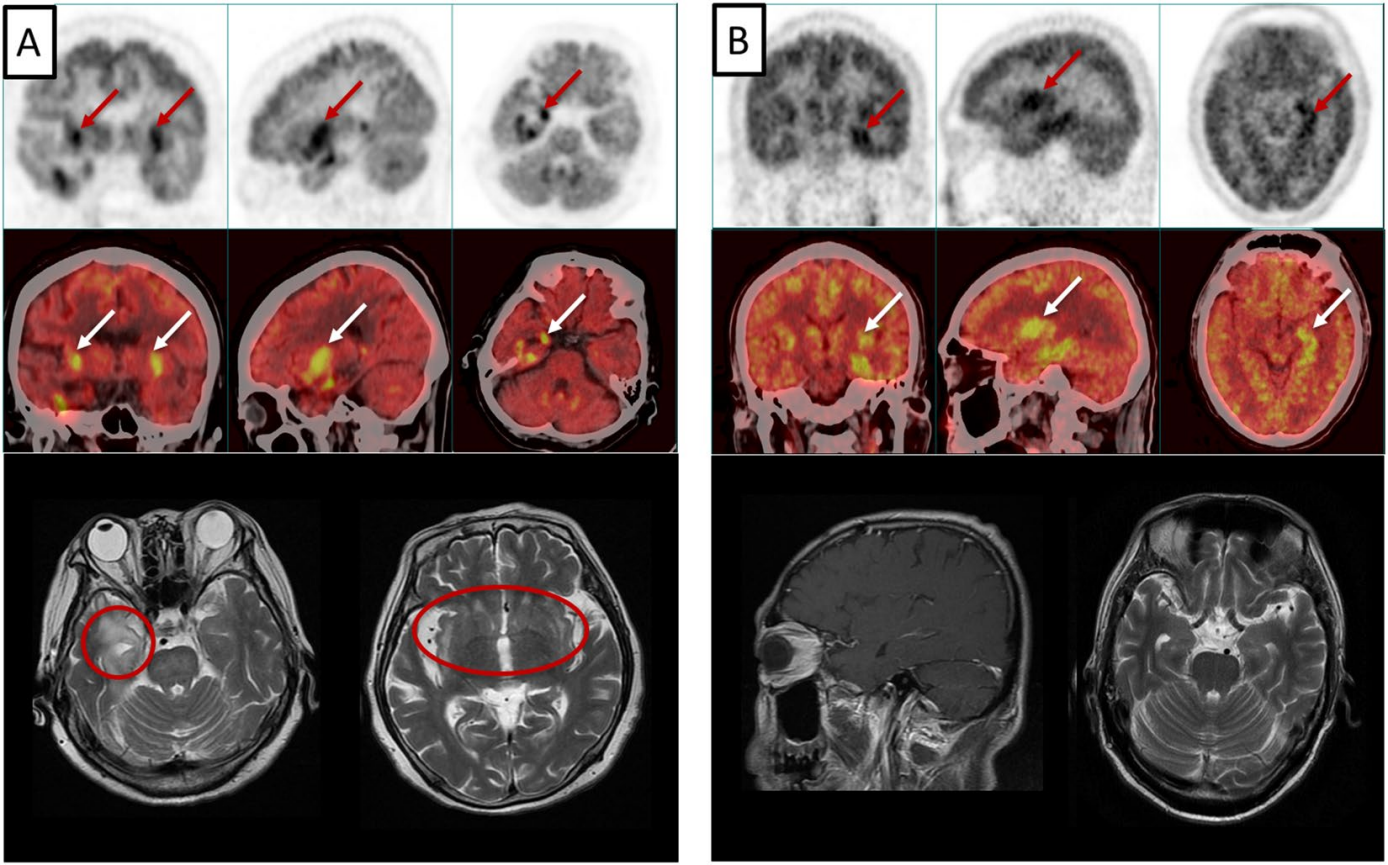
PET-CT and MRI images for two cases. Case 1: 71-year-old female (A) PET-CT showed enhanced metabolism in the bilateral mesial temporal lobe and insula (arrows), and MRI T2 showed high signal in the same location (circles). Case 2: 54-year-old male (B) PET-CT showed abnormal metabolism in the left temporal lobe (arrows), while MRI was normal.
